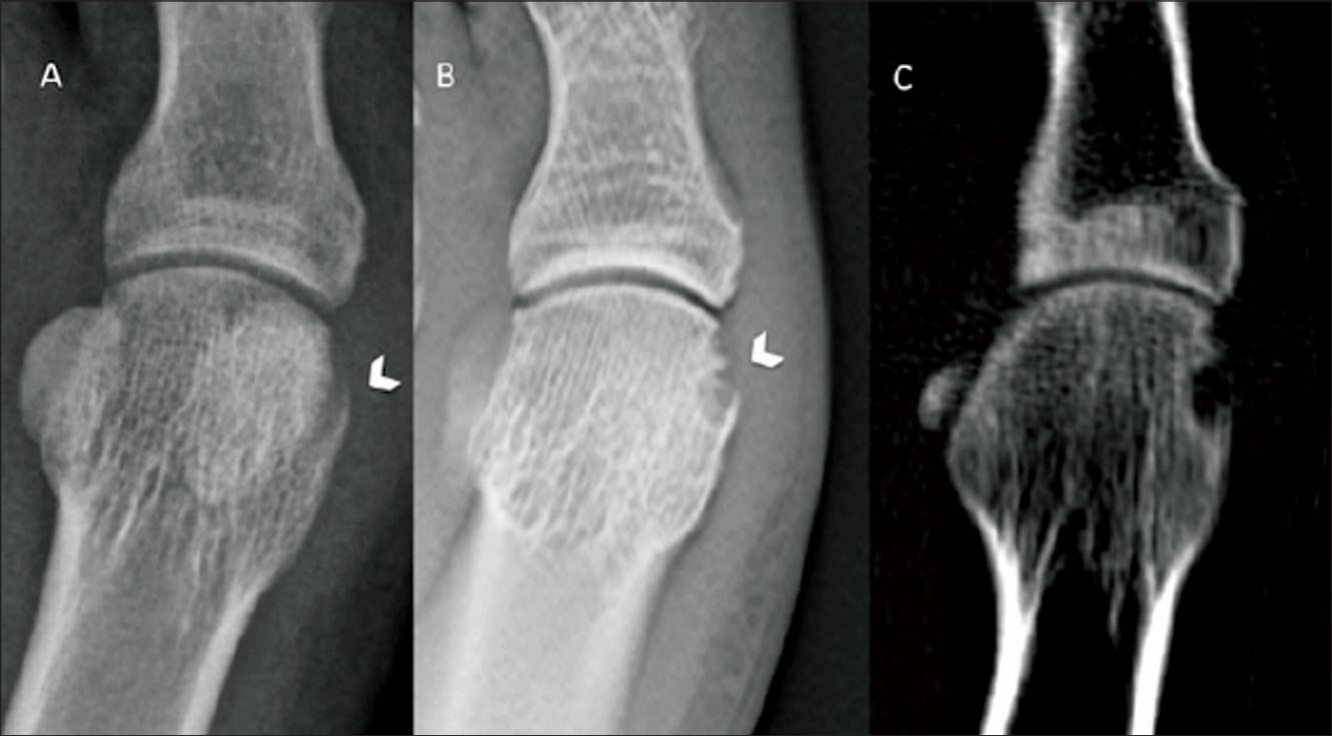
(a) A large marginal bone erosion of the medial aspect of the head of the of the first metatarsal bone (white arrowhead) is not visible on standard radiographs but (b) It is well visible on tomosynthesis (white arrowhead) and (c) It is confirmed by computed tomography (white arrowhead).
